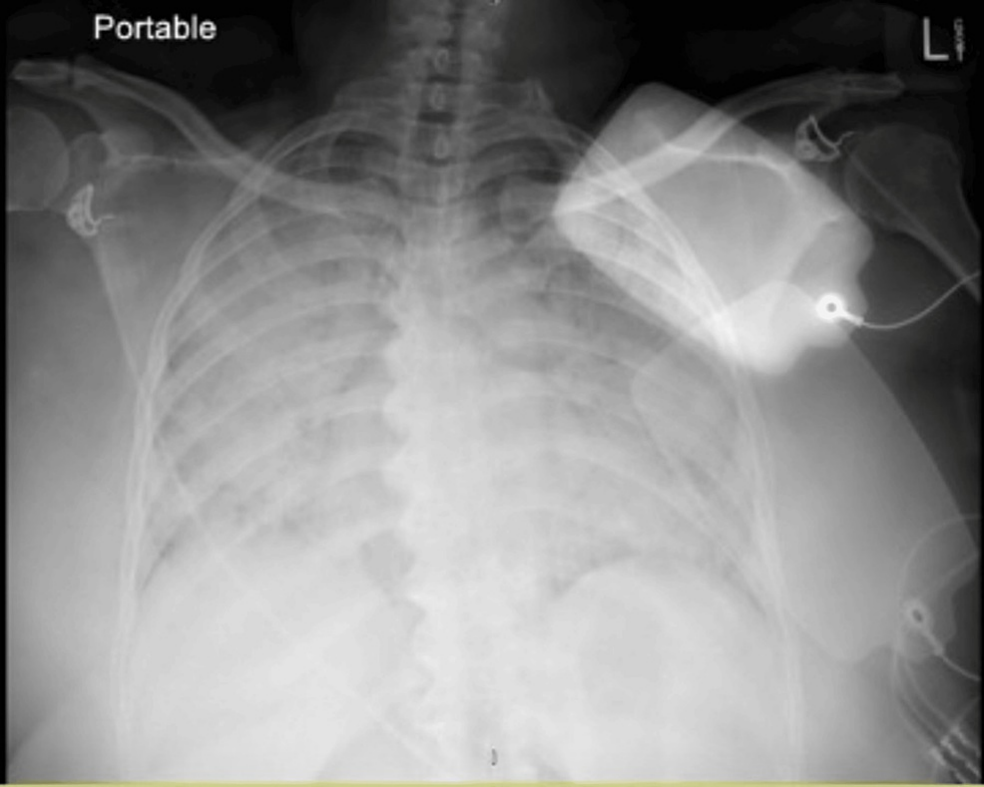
Initial chest X-ray showed bilateral diffuse opacification of the lungs
